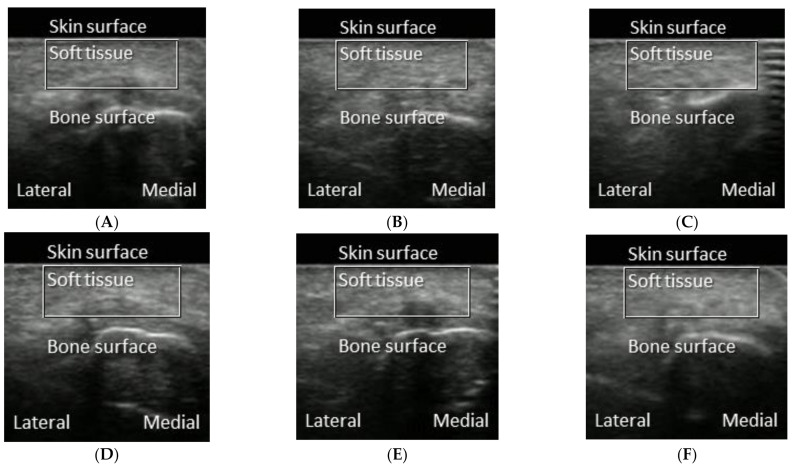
Examples of B-mode ultrasound images obtained from the first metatarsal head of a participant after different walking conditions. (A) 10 min duration at 1.8 mph. (B) 10 min duration at 3.6 mph. (C) 10 min duration at 5.4 mph. (D) 20 min duration at 1.8 mph. (E) 20 min duration at 3.6 mph. (F) 20 min duration at 5.4 mph. Each image shows the region of interest (ROI, from the surface of the skin to the surface of the first metatarsal head with a width of 100 pixels) and the medial and lateral sides of the participant.
